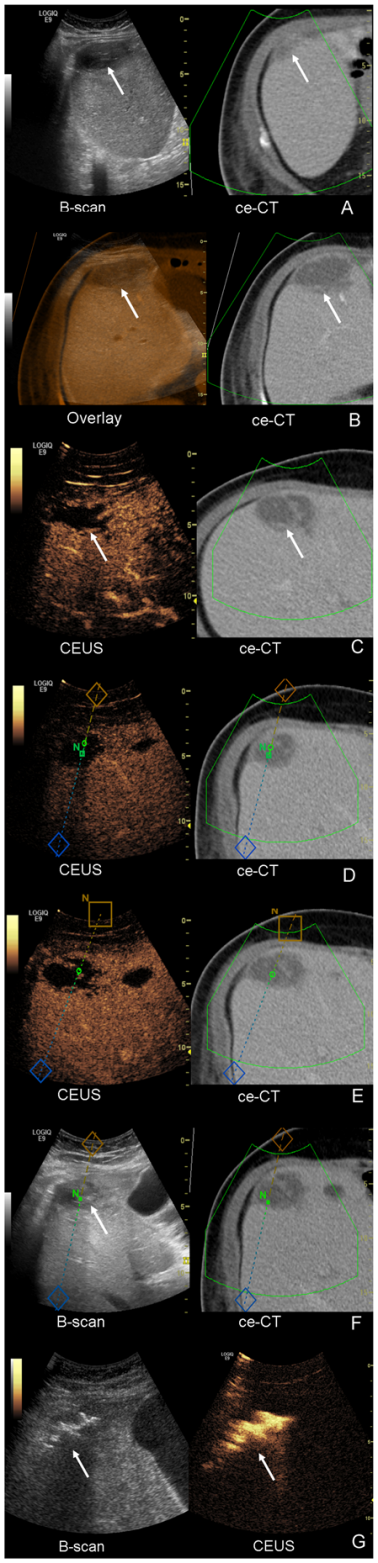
Abscess drainage performed with image fusion (ultrasound and CT). A 45 years old patient presented with elevated infection parameters and fever after partial resection of the stomach. Contrast-enhanced CT showed an abscess. The referring surgeons requested a drainage. Figure 3A. Fusion of fundamental B-Scan and contrast-enhanced CT for planning the intervention shows subcapsulary a mostly liquid formation in liver segment VIII (white arrow). Figure 3B. Overlay technique (color-coded CT and fundamental B-Scan) for optimized fusion. Figure 3C. CEUS shows a clearly demarcated mostly liquid formation in liver segment VIII and excellent image fusion with ceCT. Figure 3D. Planning of needle tracking (V Nav). The virtual puncture line is showing the anticipated end point of the needle in the middle of the formation (green circle). The subcostal cutaneous beginning of the puncture line lies outside the displayed plane (brown square marked ‘N’). Figure 3E. The needle tip now lies in the center of the formation (small green quadrant marked ‘N’) and next to the planned location (green circle). Figure 3F. B-scan control confirms needle tip within the formation (white arrow). Figure 3G. Drainage has been inserted in Seldinger technique. US contrast agent applied over drainage is documented within the abscess formation in parallel imaging (B-scan and contrast mode).
